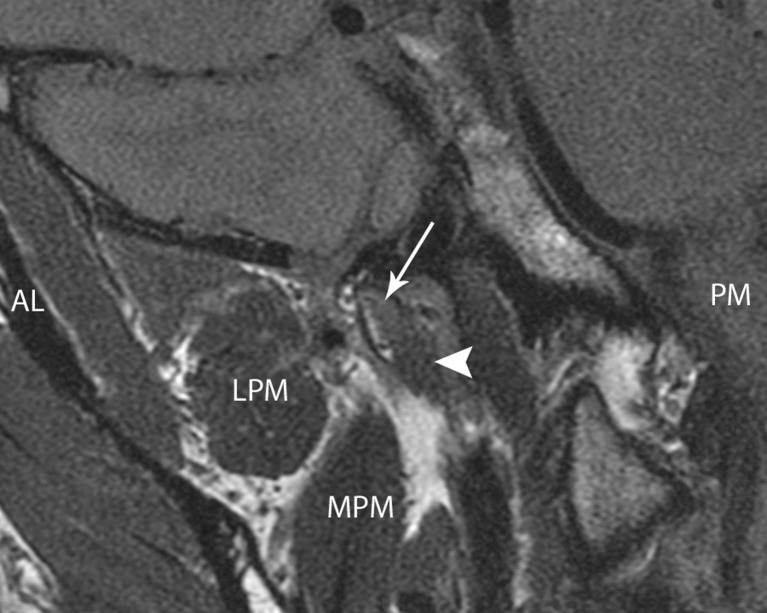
Coronal oblique proton density MRI of the right ET and paratubal structures in a 36-year-old healthy male volunteer. The tubal cartilage is indicated by the *arrow*, the levator palatini muscle by the *arrowhead. LPM* lateral pterygoid muscle. *MPM* medial pterygoid muscle. *AL* and *PM* denote antero-lateral and posteromedial orientation of the imaging plane
